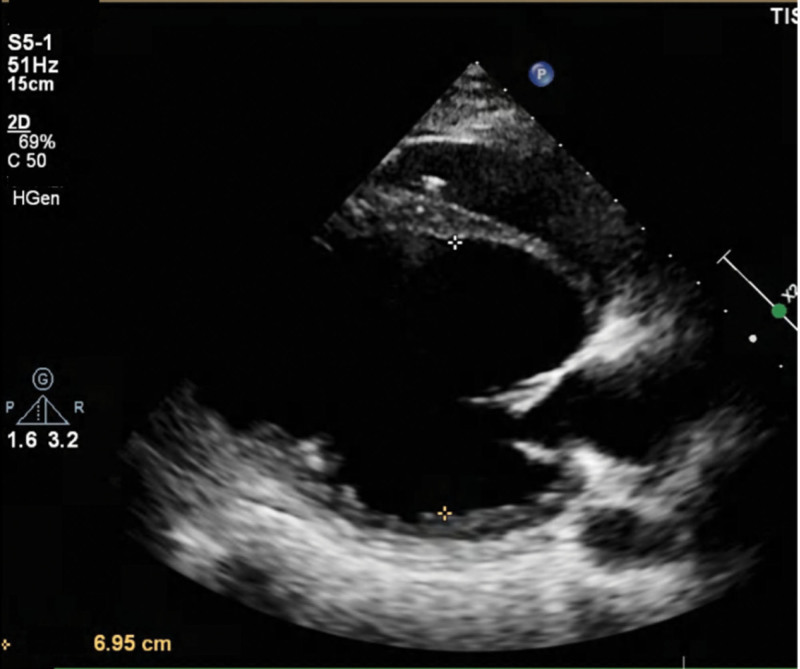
Transthoracic echocardiography. Follow-up echocardiograms demonstrated progressive improvement in cardiac function after immunoadsorption therapy. On September 14, 2024, the left atrium measured 38 mm, the left ventricle 71 mm, with moderate mitral regurgitation and an LVEF of 28%. At the external hospital follow-up on October 15, 2024, the LVEF further improved to 29.2%. LVEF = left ventricular ejection fraction.
